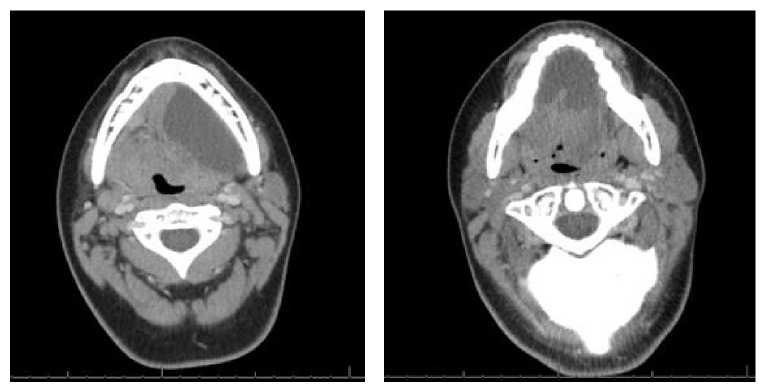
CT scan with IV contrast revealing a fluid filled, unilocular sac with extension across the midline and into the submandibular space.
